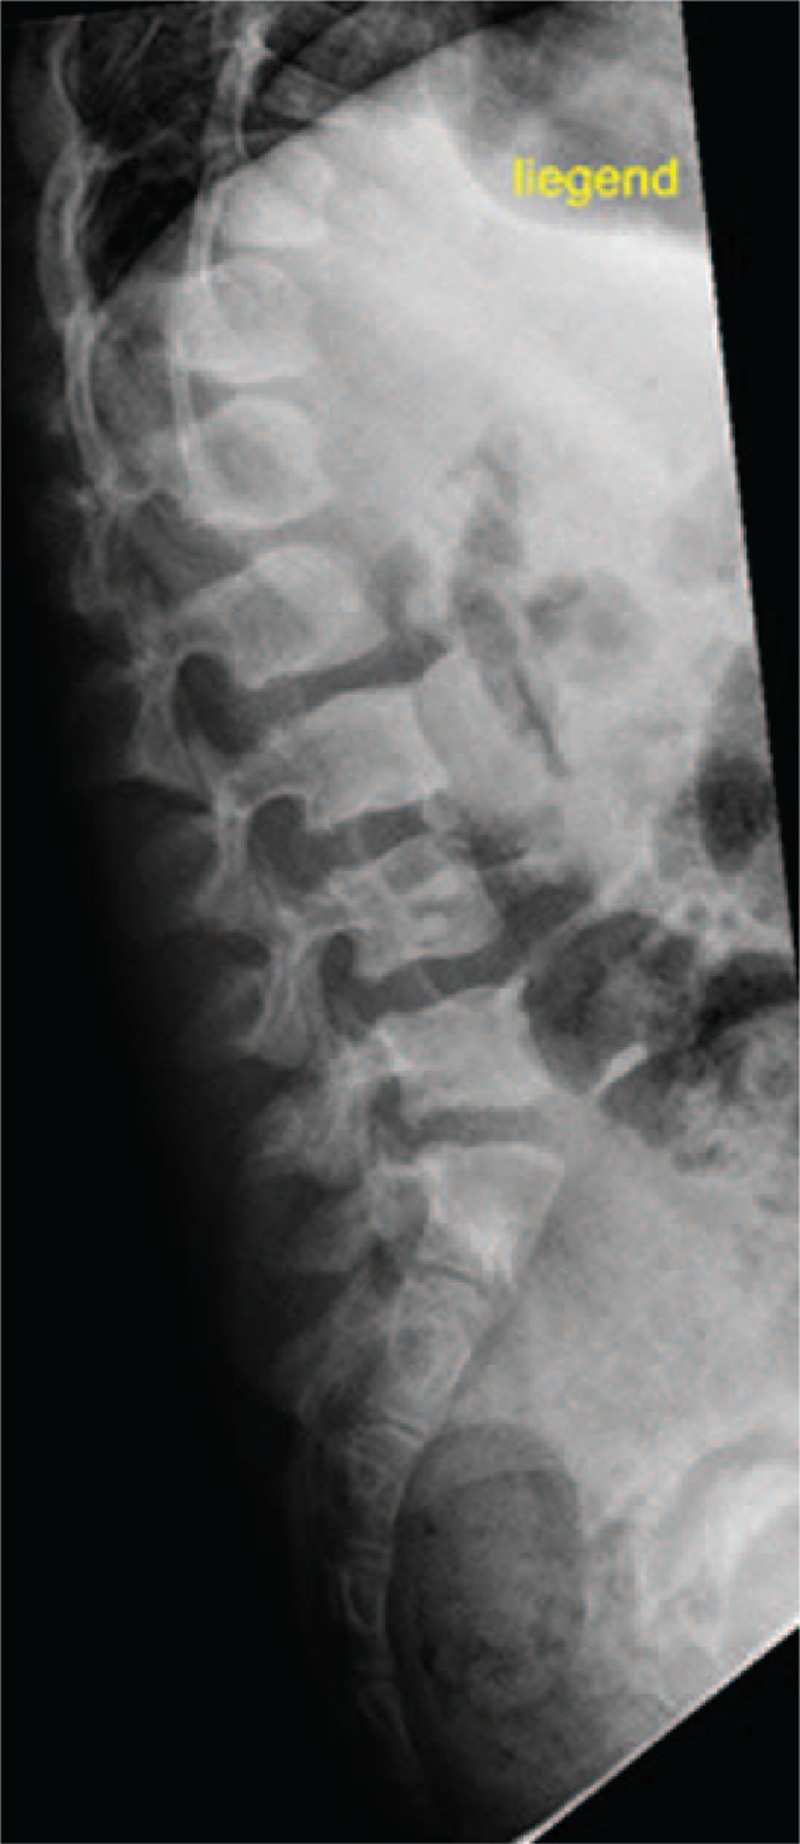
Lateral spine radiograph subject (IV,10) showed anterosuperior hypoplasia and ovoid configurations of T10 to T12. T12 showed marked posterior end plate hypoplasia and L1 to L5 showed anterior end plates concavities.
